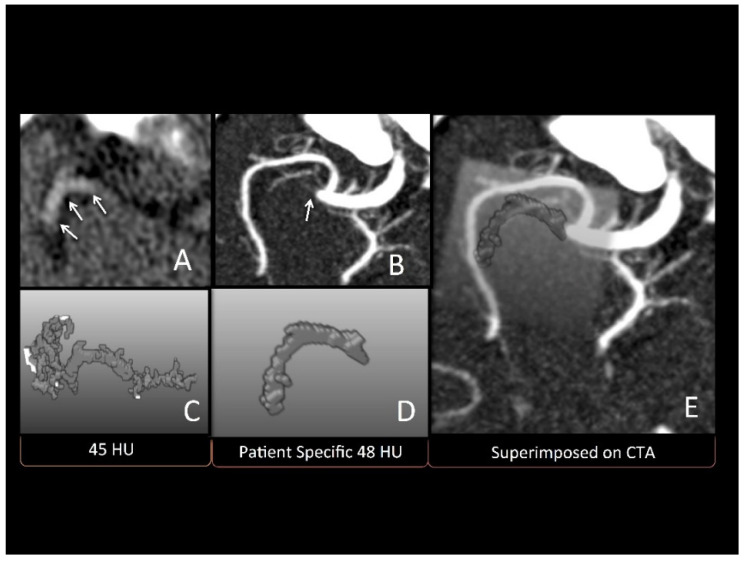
Patient with right distal middle cerebral artery M1 occlusion extending into the M2: (A) hyperdense sign in NCCT (marked by the arrow); (B) thrombus in baseline CTA; (C) three-dimensional model using conventional 45 HU threshold, which does not accurately depict CTA thrombus; (D) patient-specific threshold of 48 HU; (E) three-dimensional model using patient-specific HU threshold super-imposed onto CTA.
